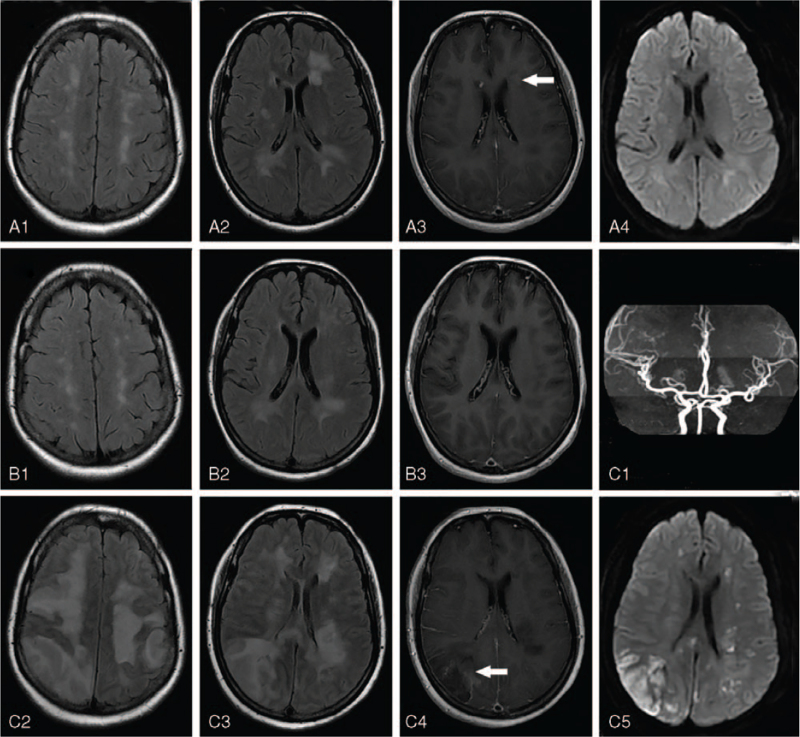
Brain magnetic resonance imaging (MRI) findings. The initial brain MRI revealed bilateral multiple hyperintense lesions in periventricular white matter, centrum semiovale, and corpus callosum on T2 fluid attenuated inversion recovery (FLAIR) images (A1, A2), while light enhancement in periventricular white matter on T1 enhanced images (A3, arrow), and dotted hyperintense lesions in diffusion weighted images (DWI) (A4). Repeated brain MRI showed the lesions decreased significantly at 2 months after discharge (B1, B2, B3). The latest brain MRI showed the lesions were enlarged and increased on T2 FLAIR (C2, C3) and DWI (C5), and the right occipital lobe lesion had open-ring enhancement on T1 enhanced images (C4, arrow). Brain magnetic resonance angiography (MRA) was normal (C1).
